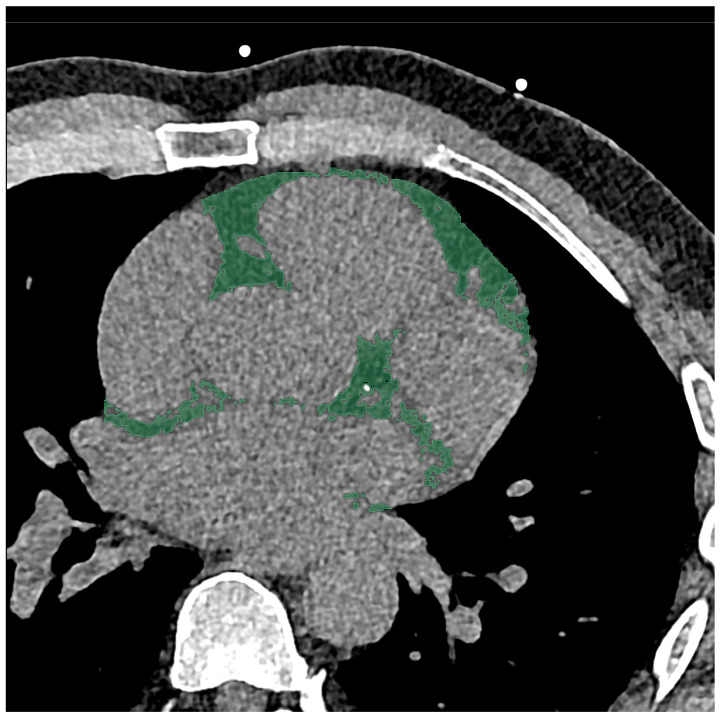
Segmentation of the epicardial adipose tissue was performed on an axial view CT scan with a slice thickness of 2 mm. On this scan of a 55-year-old male, the area of segmentation is marked in green.
